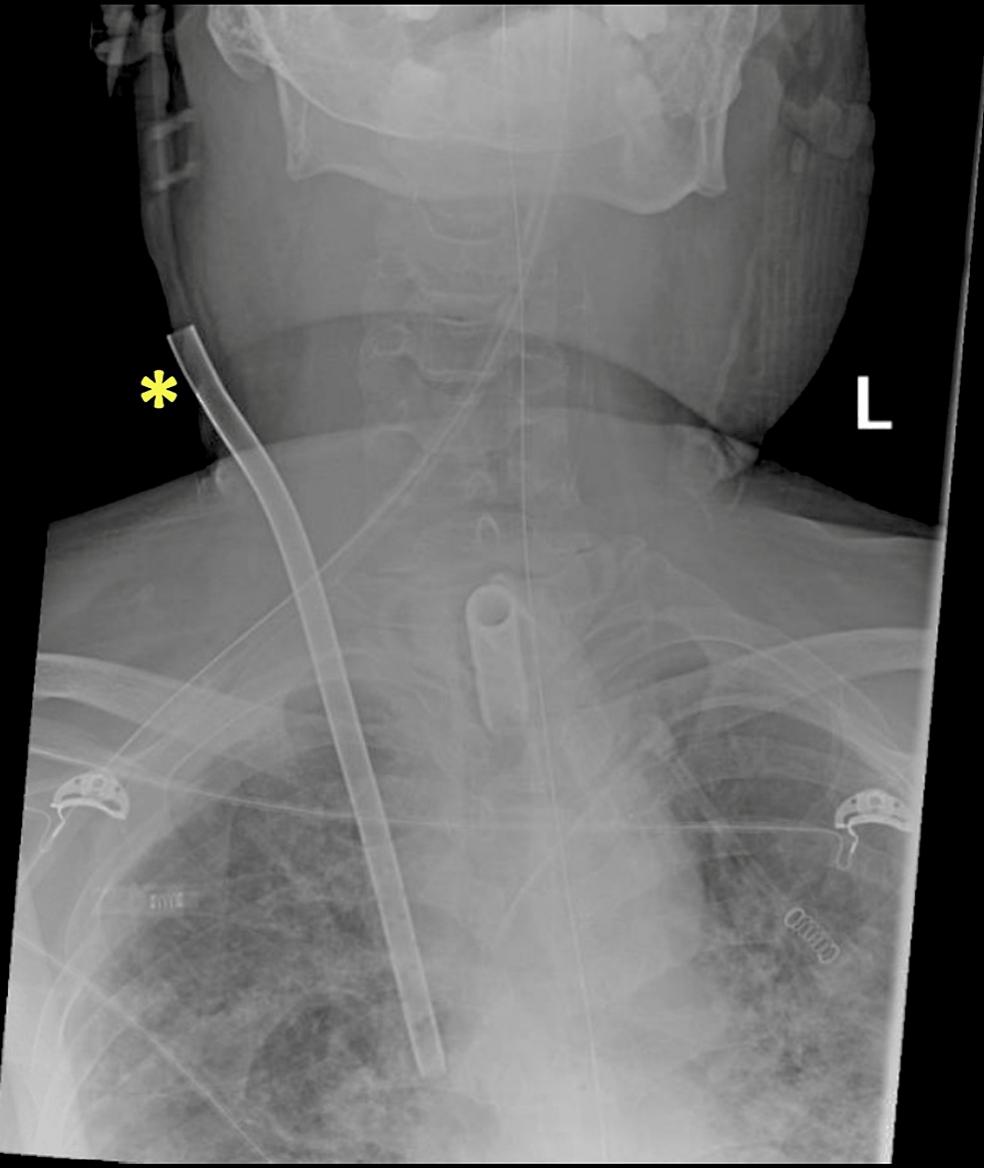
Anteroposterior radiograph of the head, neck, and chest. The yellow asterisk denotes the extracorporeal membrane oxygenation catheter.
